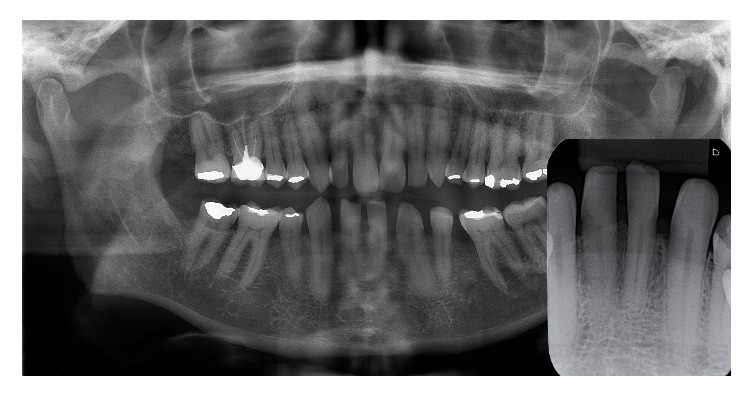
Panoramal radiograph showing agenesis of the lower lateral incisors and premolars. Inset periapical radiograph showing no evidence of bone involvement.
